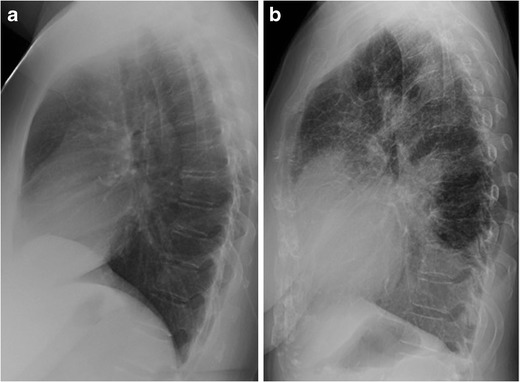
Lateral chest radiographs demonstrating a normal retrosternal clear space (a), the lucent area between the sternum and ascending aorta, and retrosternal reticular opacities due to interstitial pulmonary oedema, known as “Kerley D lines” (b). Pleural effusions accompany the oedema
